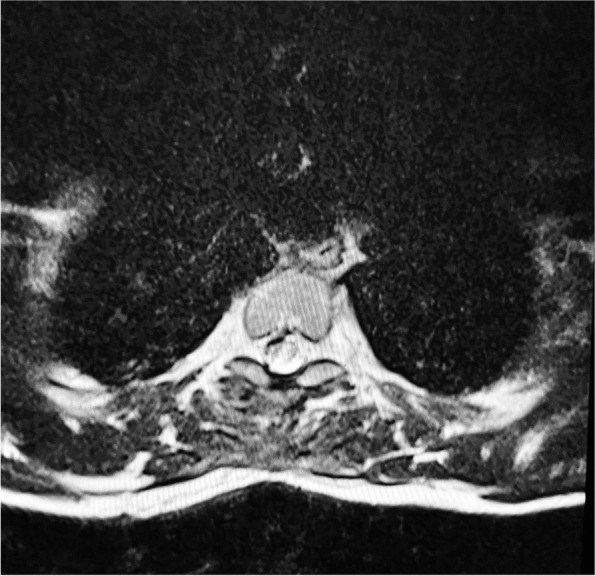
Three month Post-Operative MRI Axial T2 weighted MRI at T2/3 level demonstrating the cord high signal in the repaired area with evidence of healing
